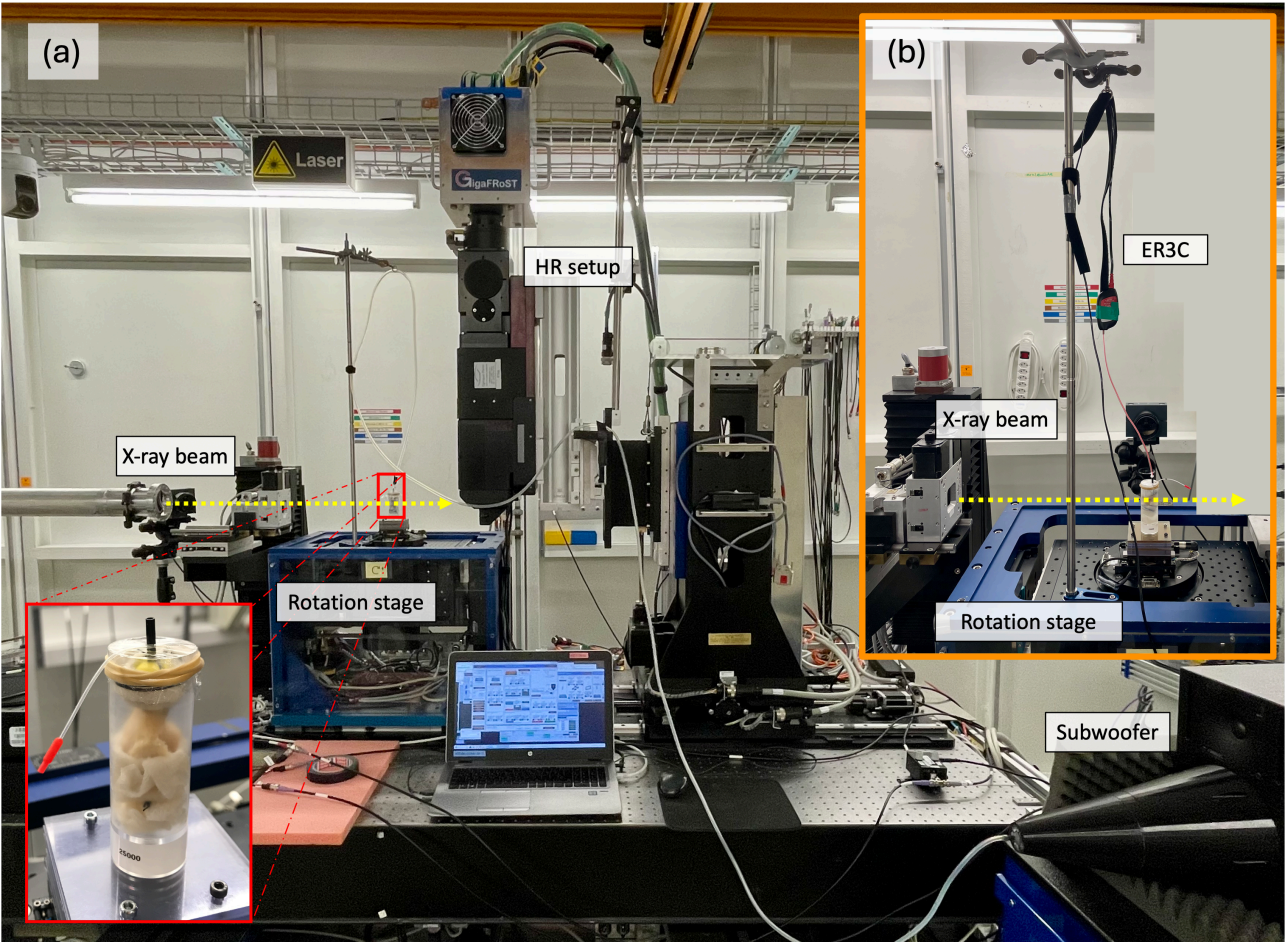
Experimental setup at the TOMCAT beamline. The sample is wrapped in saline-soaked neuro-patties to keep it from drying, fixed in a custom-made sample holder, and mounted onto the rotation stage. The top is sealed with a plastic film, showing two tubes coming out (see zoom-in, bottom left of (a)). The black one is the tip of the earplug, which is sewn on one end to the external ear canal and connected over a silicone tube to the subwoofer (a) or to ER3C Insert Earphones from Etymotic coupled to an amplifier (b) on the other hand. The transparent tube with the red tip is inserted into the probe microphone during calibration. The sample is fixed onto the rotation stage. The X-ray beam comes from the left (indicated with a yellow arrow). The HR setup consists of the in-house built GigaFRoST camera [26] and a 4x magnification high numerical aperture macroscope from Optique Peter [27].
